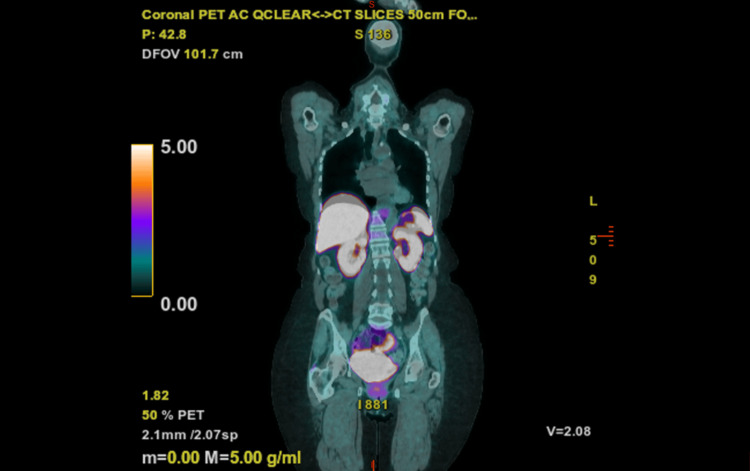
Representative image from 68Ga-DOTATATE PET/CT scan demonstrating no evidence of somatostatin receptor-positive tumors
